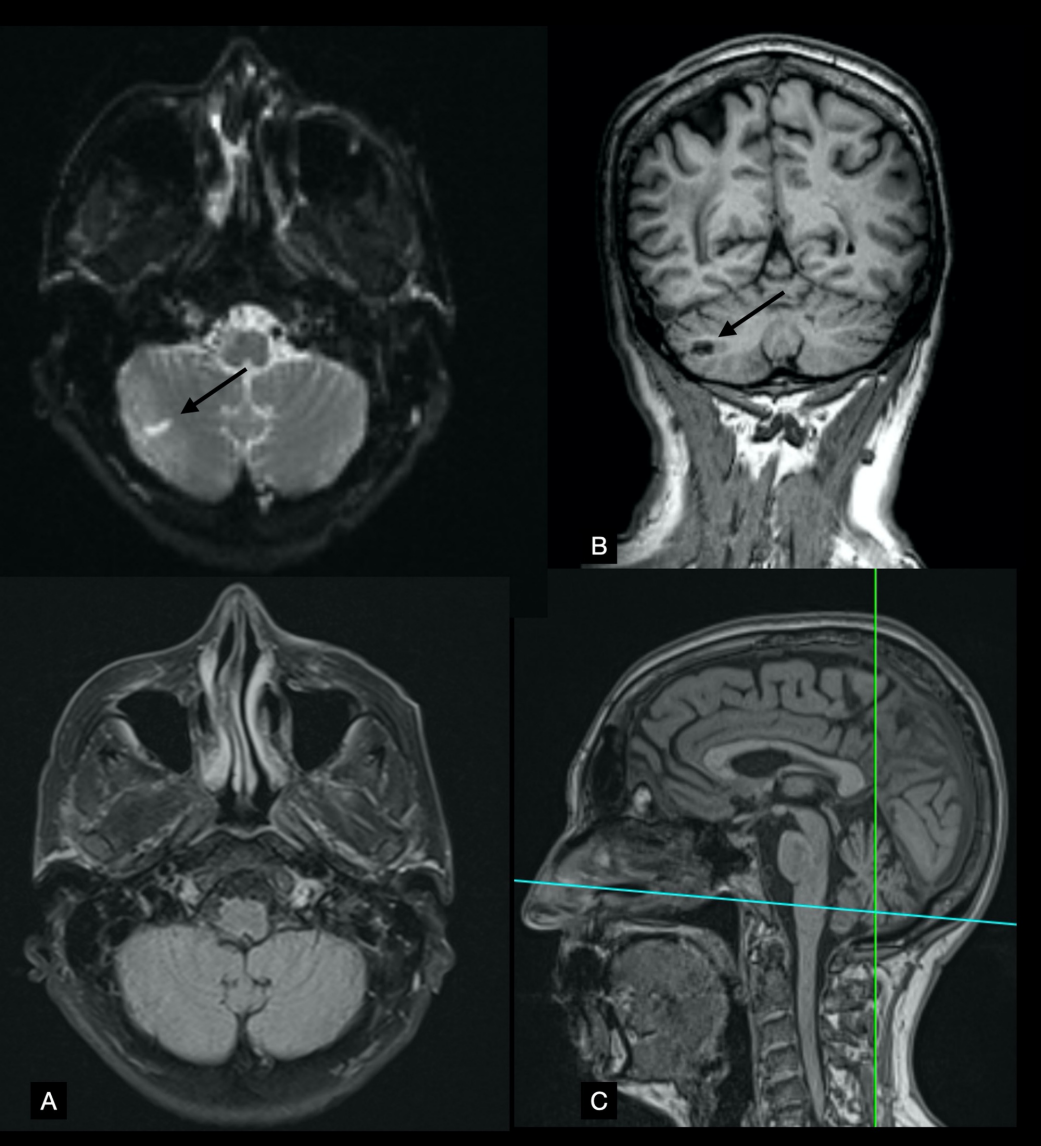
Cerebellum MRI Panel A shows axial diffusion-weighted imaging (DWI) (top) and fluid-attenuated inversion recovery (FLAIR) imaging (bottom) at the level of the medulla. Panel B shows the same lesion as panel A (black arrows) on a T1-weighted coronal plane. Panel C shows the positions of A (horizontal, blue line) and B (vertical, green line) on a T1-weighted image of the midline sagittal plane.
